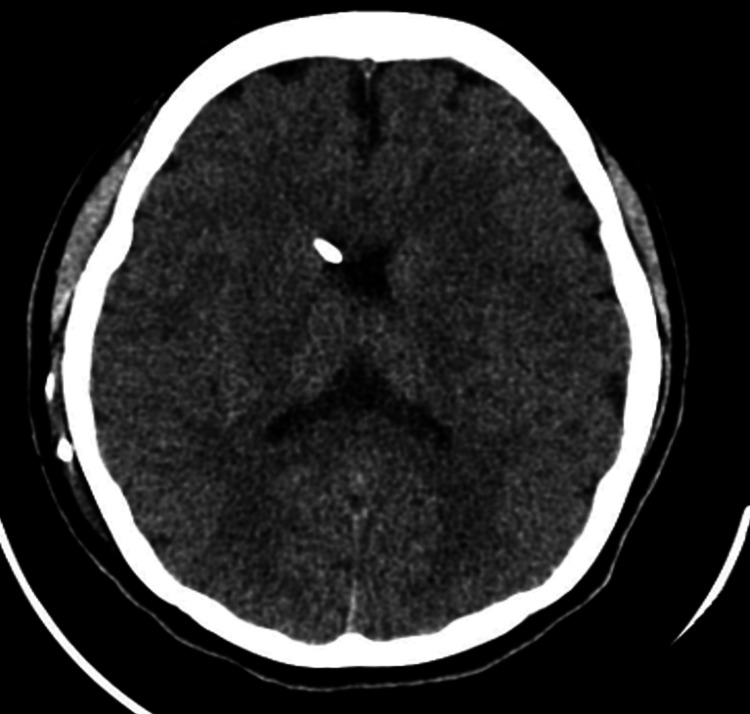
CT brain (axial view) showing ventricles of normal size with a VPS in situ. CT: computed tomography; VPS: ventriculoperitoneal shunt
